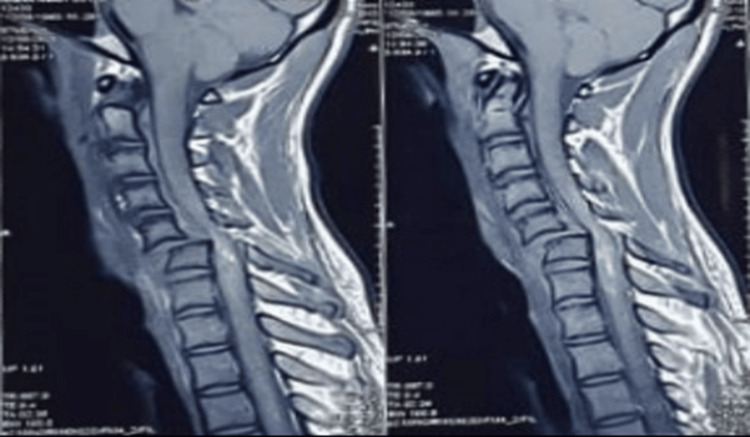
MRI of cervical spine Cervical spine MRI showing the fracture dislocation at the level C5 and C6 vertebra with compression to the spinal cord at that level.
